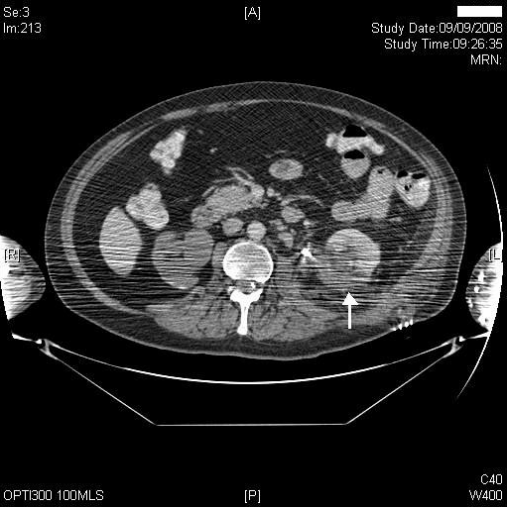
CT of kidneys, performed on 09 September 2008 (arterial phase), showed non-perfusion in posterior cortex of left kidney (arrow); left ureteric stent in place; marked hydronephrosis of right kidney.
